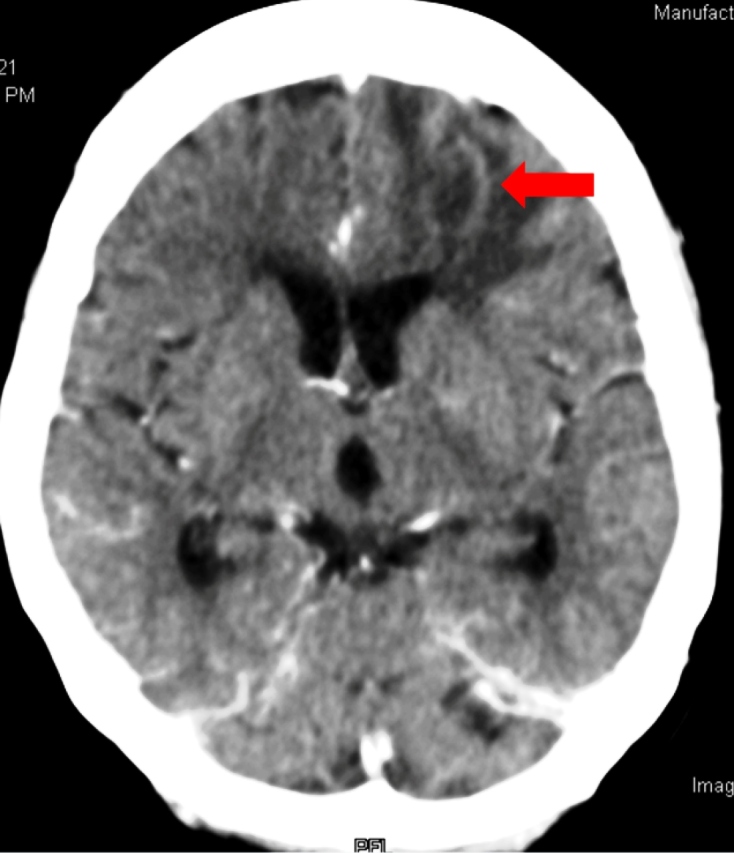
Computed tomographic scan of the brain showing abscess in the left frontal lobe (arrow).
